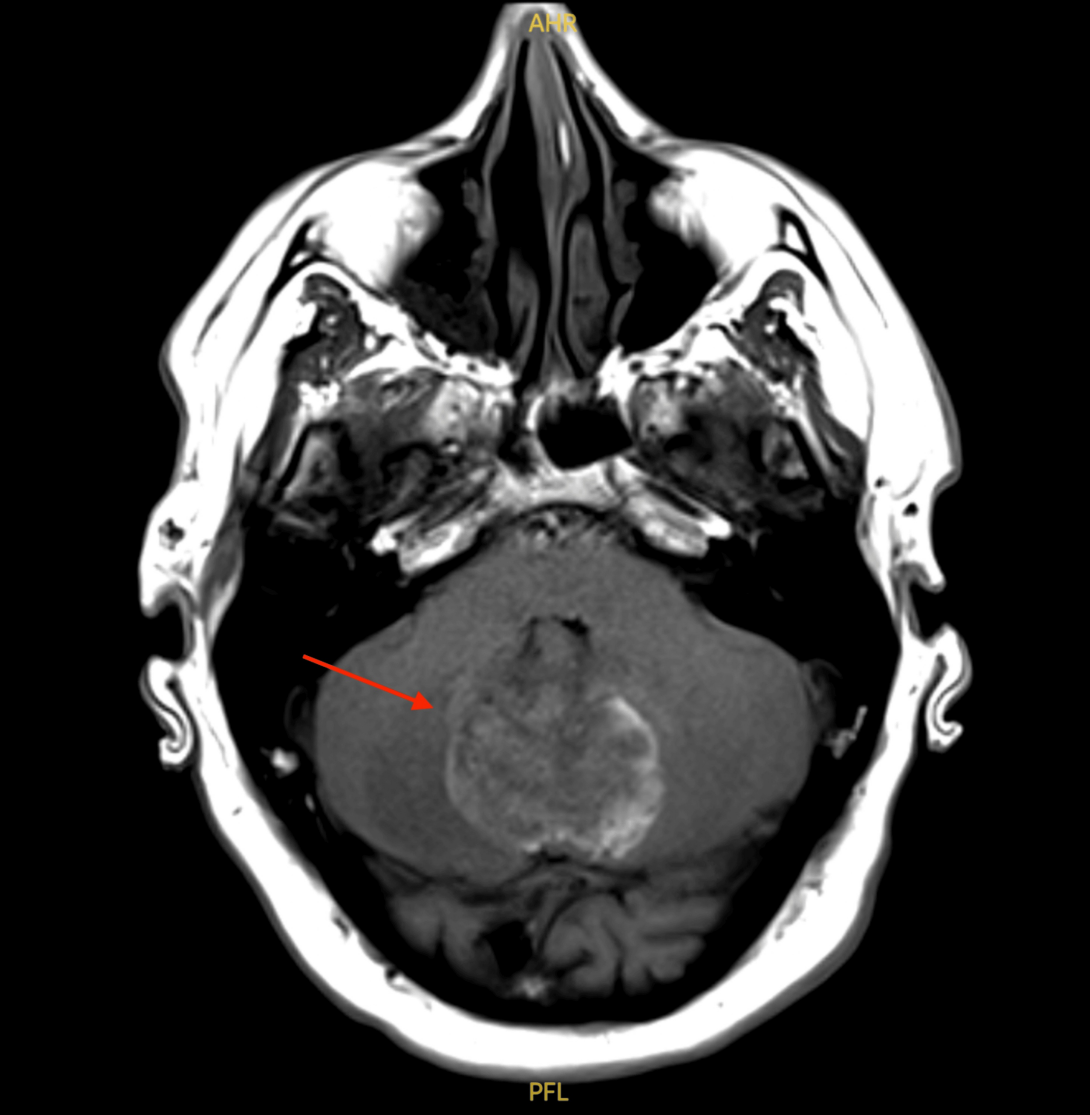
Axial post-contrast MRI showing a 4.4 cm hemorrhagic midline posterior fossa mass (red arrow) with mild enhancement and mass effect on the fourth ventricle Anterior horn of lateral ventricle (AHR) and posterior fossa lesion (PFL) are annotated in the image. Image Credit: Daniel Jones
